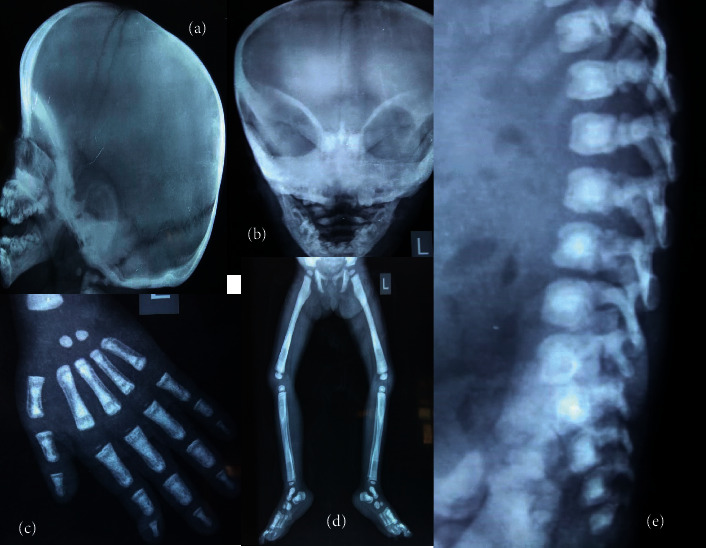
(a) & (b) X-rays in case 4: Skull lateral and AP view showing calvarian and basilar thickening, poorly developed sinuses and sclerosis of nasal bone; (c) X-ray hand showing bone in bone appearance; (d) X-ray lower limbs showing bone in bone appearance and increased bone density; (e) X-ray spine lateral view showing bone in bone appearance; (f) X-ray chest showing increased bone density in ribs and absence of corticomedullary differentiation.
